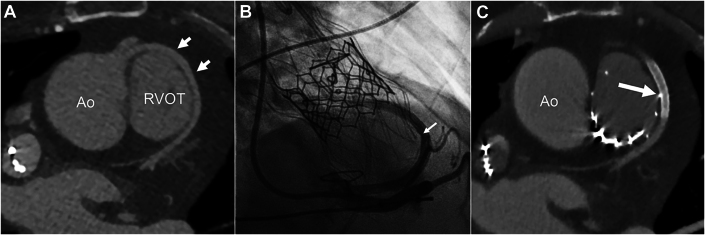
Patient 2 (A) This image from the preoperative computed tomography scan in patient 2 demonstrates the anomalous circumflex coronary artery originating from the right coronary artery and wrapping around the right ventricular outflow tract (RVOT) anteriorly (arrows). (B) This selective right coronary angiogram after placement of the Alterra Adaptive Prestent and SAPIEN 3 valve demonstrates focal compression of the anomalous circumflex artery by a proximal tine of the Prestent (small arrow). (C) This post-catheterization computed tomography image demonstrates a proximal tine of the Alterra Present projecting through the pulmonary artery and coming into contact with the stented anomalous circumflex (arrow). The portion of the coronary artery compressed by the tine now had covered stent material within it and was unobstructed. Ao = aorta.
